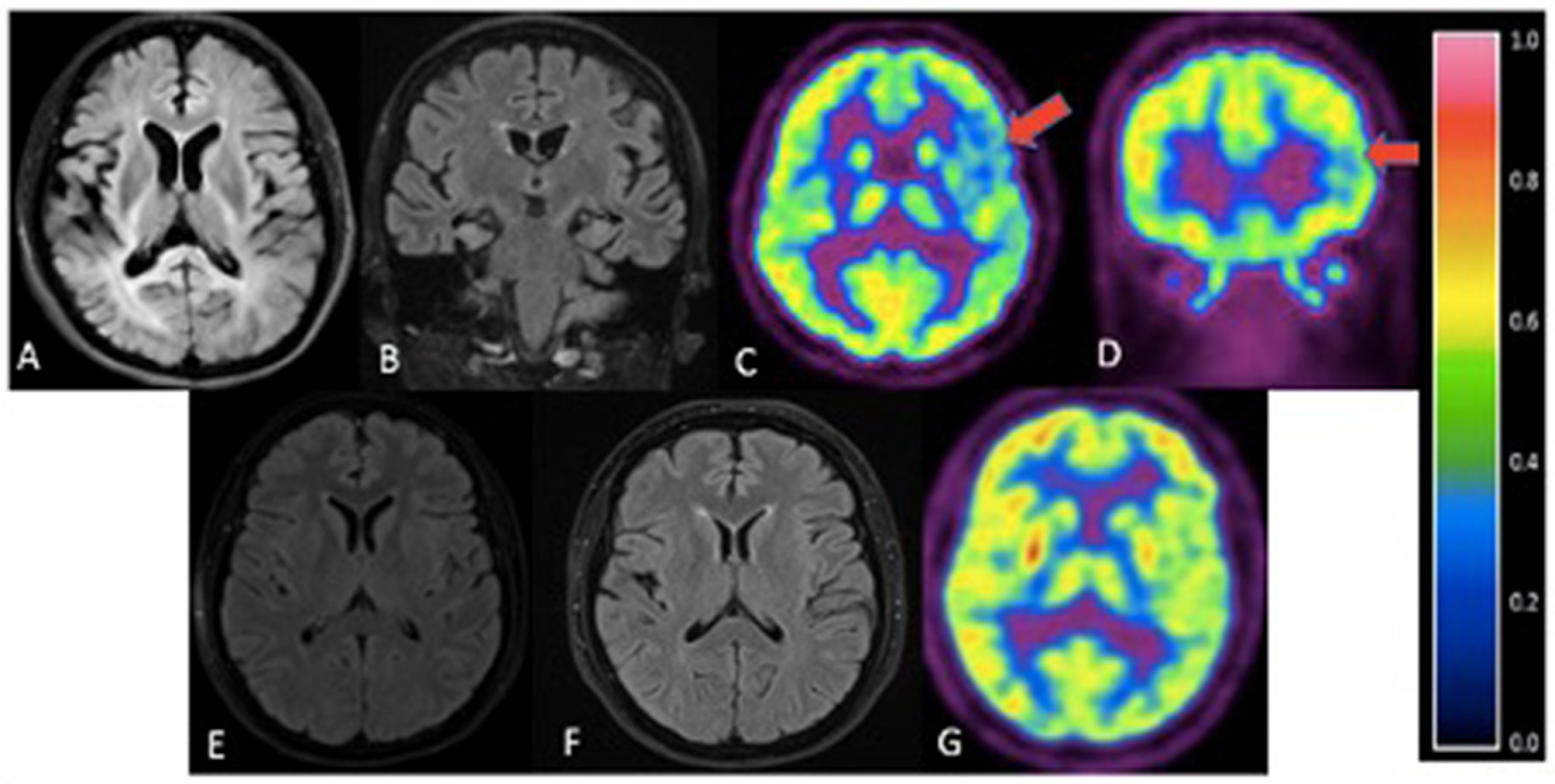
Cranial imaging of family members. (A) - III-10. Axial FLAIR. Mild atrophy of both temporal lobes. (B) - III-8. Coronal FLAIR MRI. Mild atrophy of bilateral parietotemporal areas. (C,D) III-8. Axial (C) and coronal (D) FDG-PET. Mild diffuse FDG hypometabolism in the left parietotemporal cortex (red arrows) with reference color scale to signify FDG uptake. (E) - III-6. Axial FLAIR. Unremarkable MRI. (F) - III-7. Axial FLAIR. No significant atrophy or hyperintensities. (G) - III-7. FDG-PET scan revealed no definite focus of abnormally increased or decreased FDG uptake.
